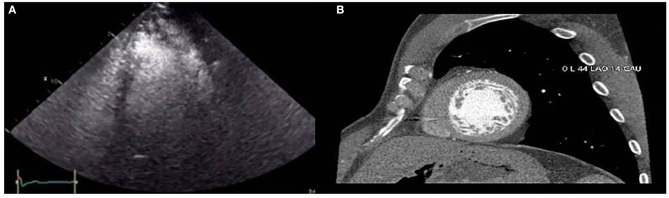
(A) Contrast echocardiography showing left ventricular non-compaction. (B) Hypertrabeculated left ventricle in one slice of the short axis view by coronary computed tomography angiography.
